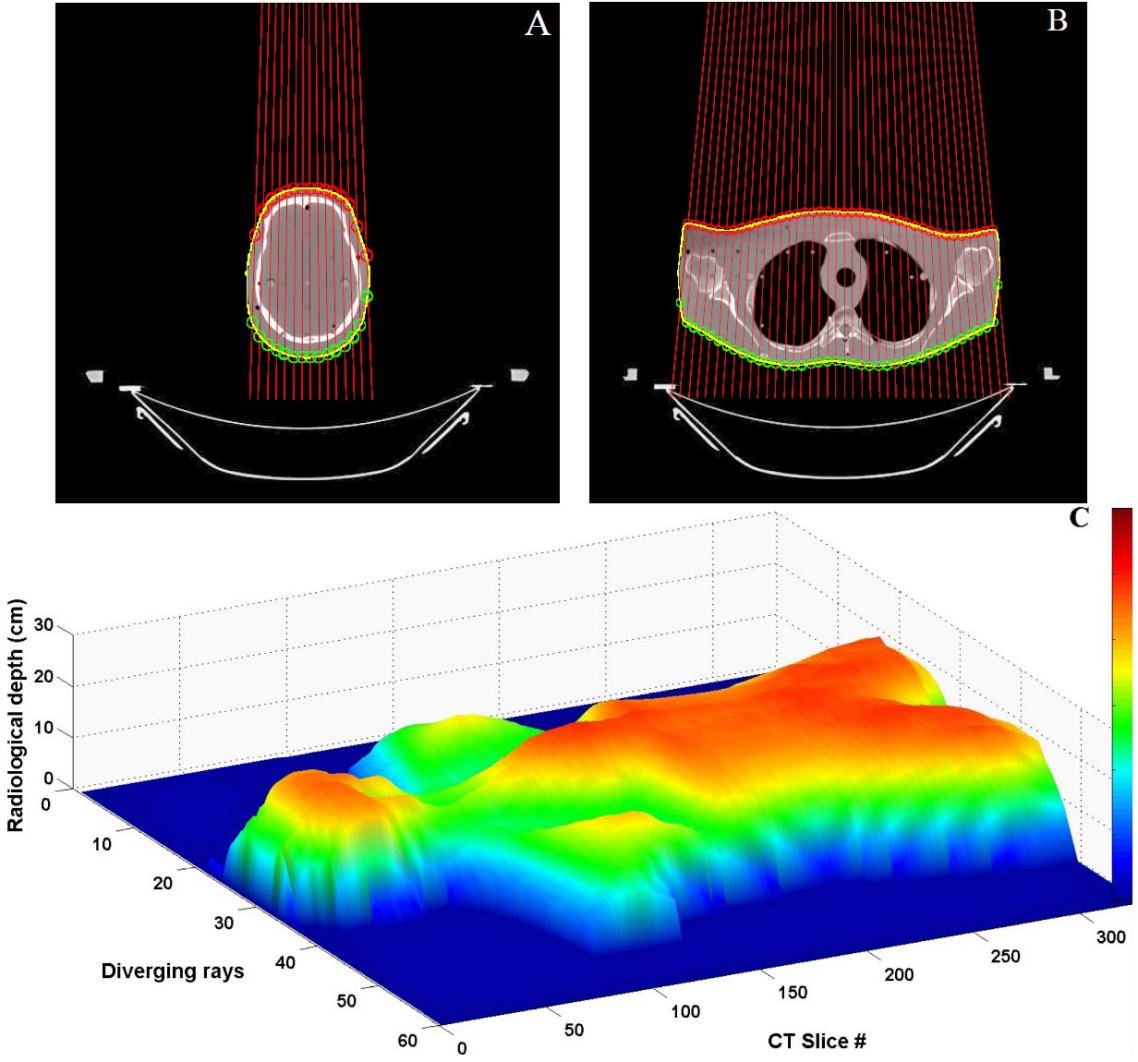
Diverging rays through CT slices at (A) head and (B) lung position. The contours are represented by yellow lines. The red and green circles indicate the entrance and exit points, respectively. Radiological depth map (C) for the entire RANDO, AP view only.
